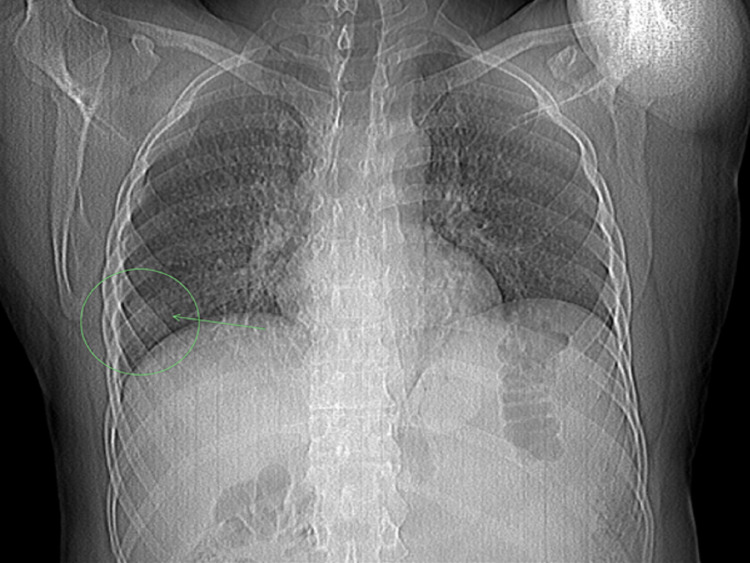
Chest X-ray. Demonstrates a well-defined nodule in the right lower lobe accompanied by a surrounding ground-glass opacity.
